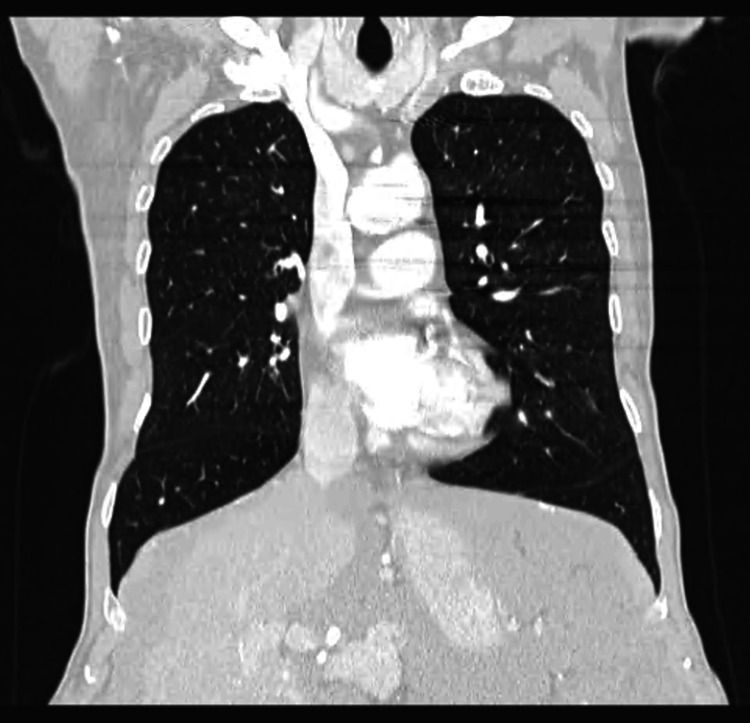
Thoracic CT scan (coronal view). No suspicious lesions were observed.
